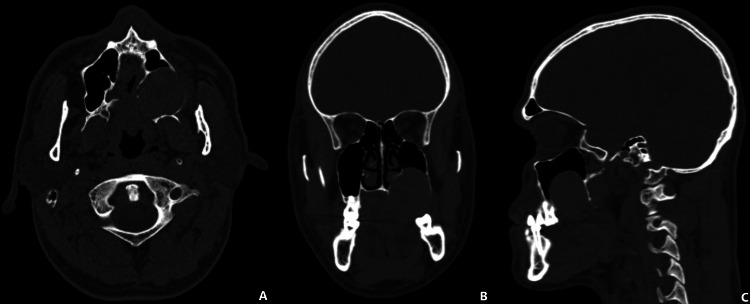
CT of the facial skeleton (A) Axial section showing extension of the lesion to the palatal midline. (B) Coronal section demonstrating expansion of the lateral wall of the maxillary sinus and extension toward the nasal fossa. (C) Sagittal section showing the posterior limit of the lesion at the pterygoid process.
